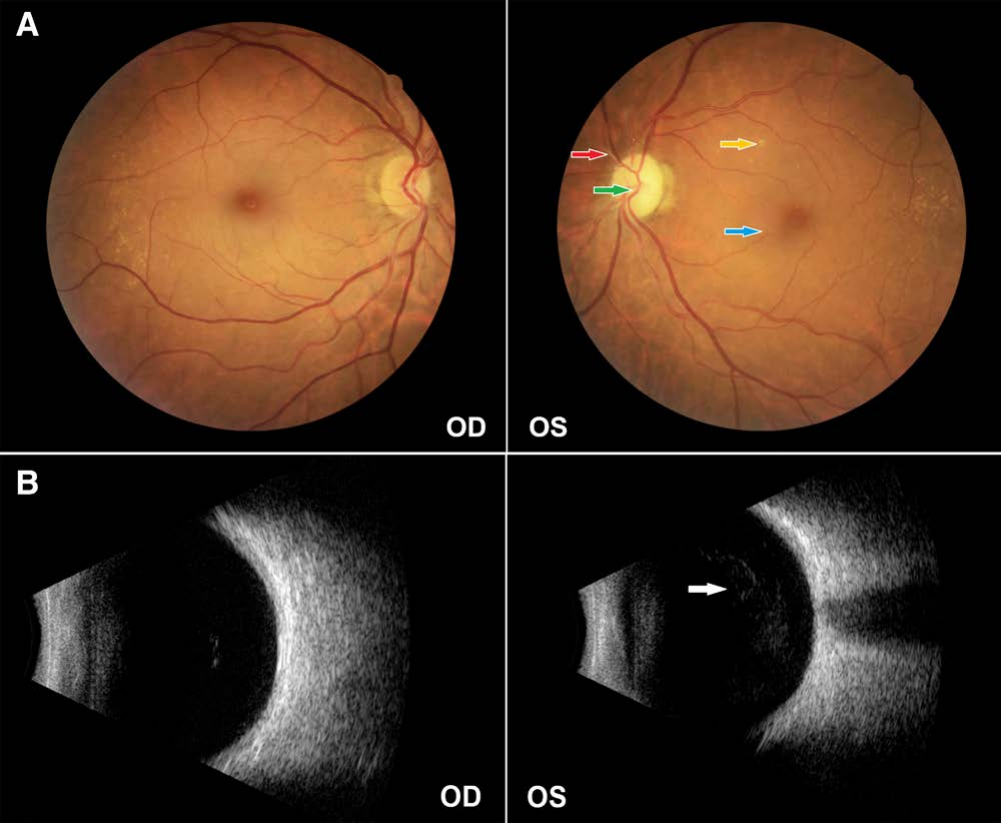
Ocular examination findings at diagnosis. (A) Fundus photographs of the right eye (OD) and left eye (OS). OD: unremarkable. OS: shows optic disc pallor (green arrow), arterial light reflex enhancement, sheathing along the superonasal branch retinal artery (red arrow), and arteriovenous crossing indentations. Multiple yellowish-white exudates are present throughout the retina (yellow arrow). Note retinal edema at the posterior pole and poor foveal reflex (blue arrow). (B) Ocular B-scan ultrasonography of both eyes. OD: within normal limits. OS: shows vitreous opacities (white arrow), suggestive of inflammatory exudates, and features consistent with optic nerve atrophy. OD = oculus dexter (right eye), OS = oculus sinister (left eye).
